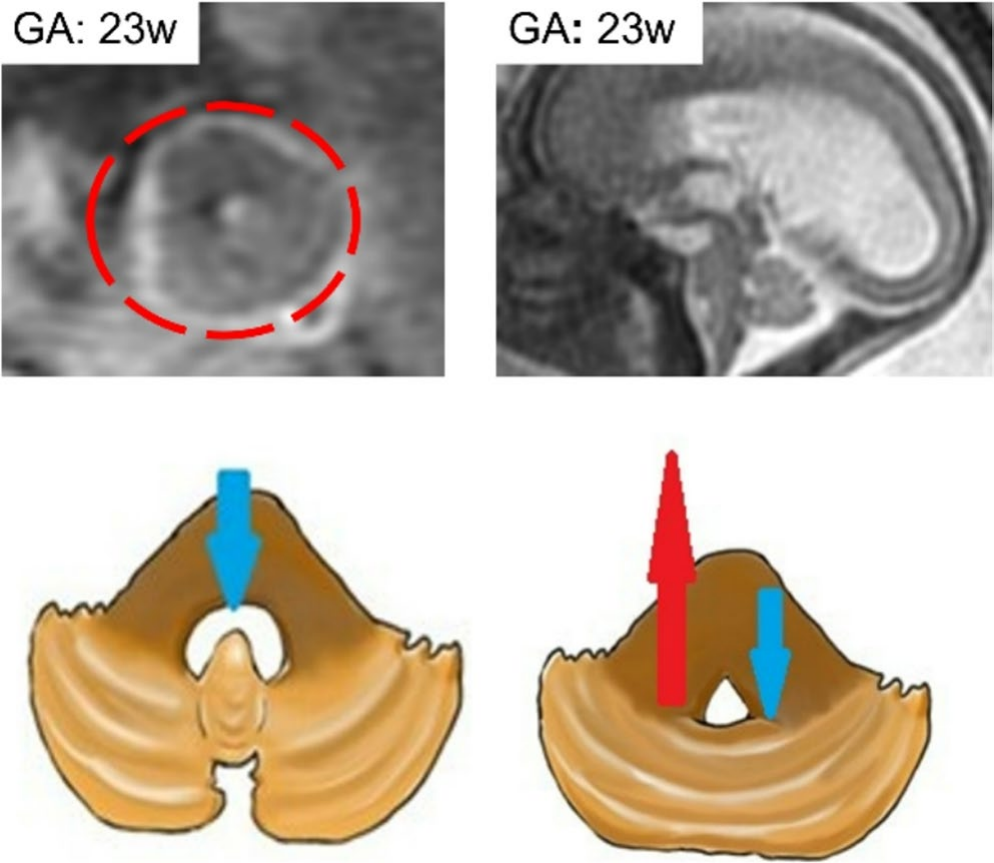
Axial and sagittal fast spin-echo T2w MR images of a 23-week fetus depict a typical agenesis of the vermis with fusion of the cerebellar hemispheres assuming a characteristic «keyhole» shape of fourth ventricle; this condition causes CSF reflux with hydrocephalus
